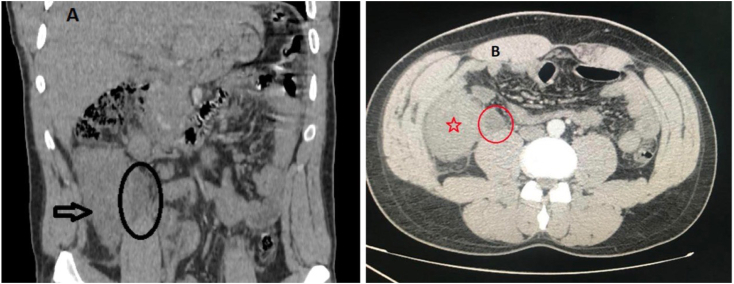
A. CT scan of the abdomen (coronal plane) B. CT scan of abdomen (axial plane) reveal an enlarged appendix (encircled black circle and red circle) and fluid. (For interpretation of the references to colour in this figure legend, the reader is referred to the Web version of this article.)
